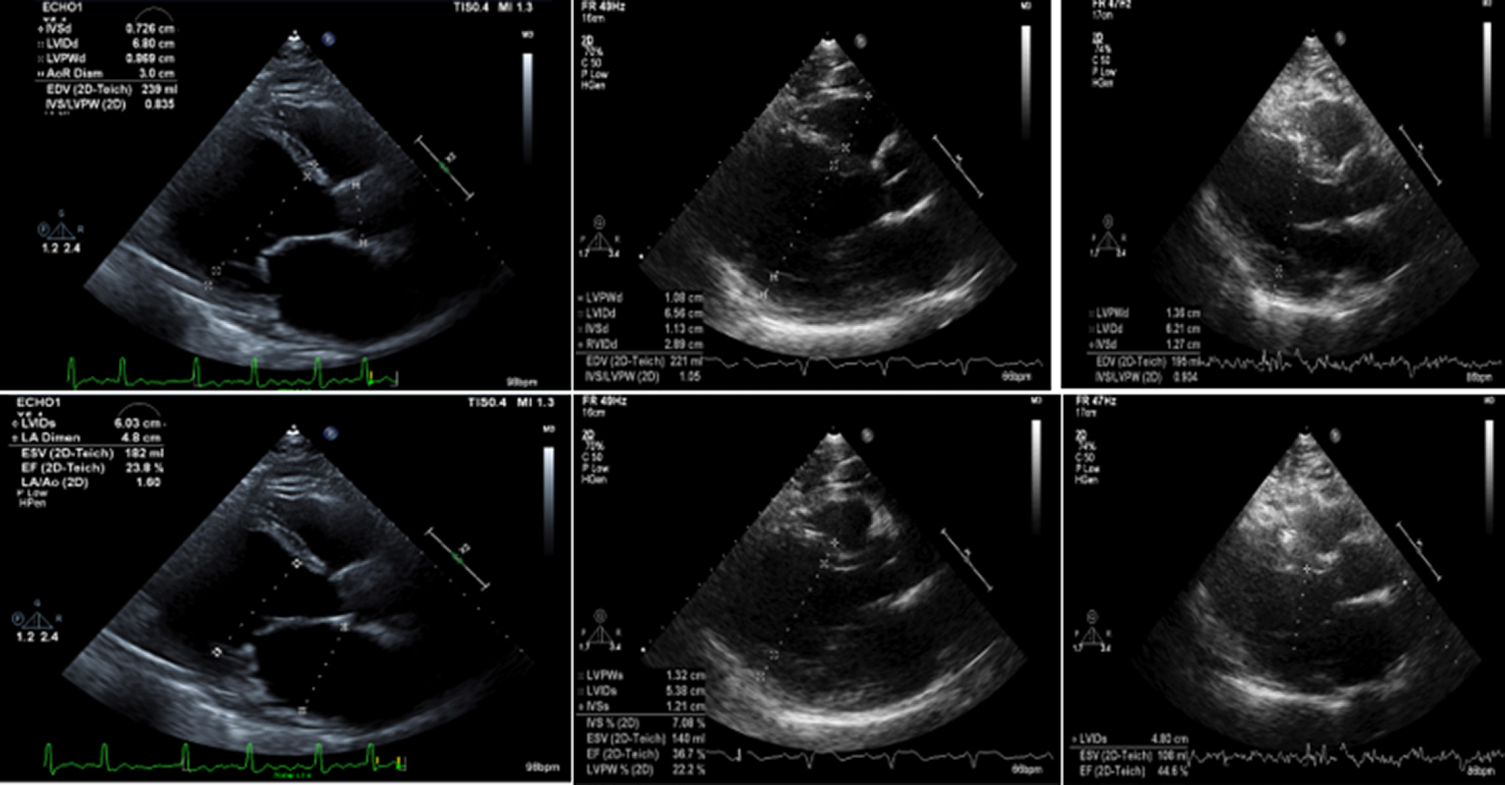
Echocardiogram showing severely reduced LVEF and dilated Left ventricle and atria on the left panel (on admission) with subsequent improvement of LVEF to 44% (at 12 months) on the right panel. The middle panel of echo was obtained at 3 months showing improvement in LVEF and heart dimensions. The upper panels of images were obtained at end‐diastole and lower panels at end‐systole. 563 × 304 mm (38 × 38 DPI)
